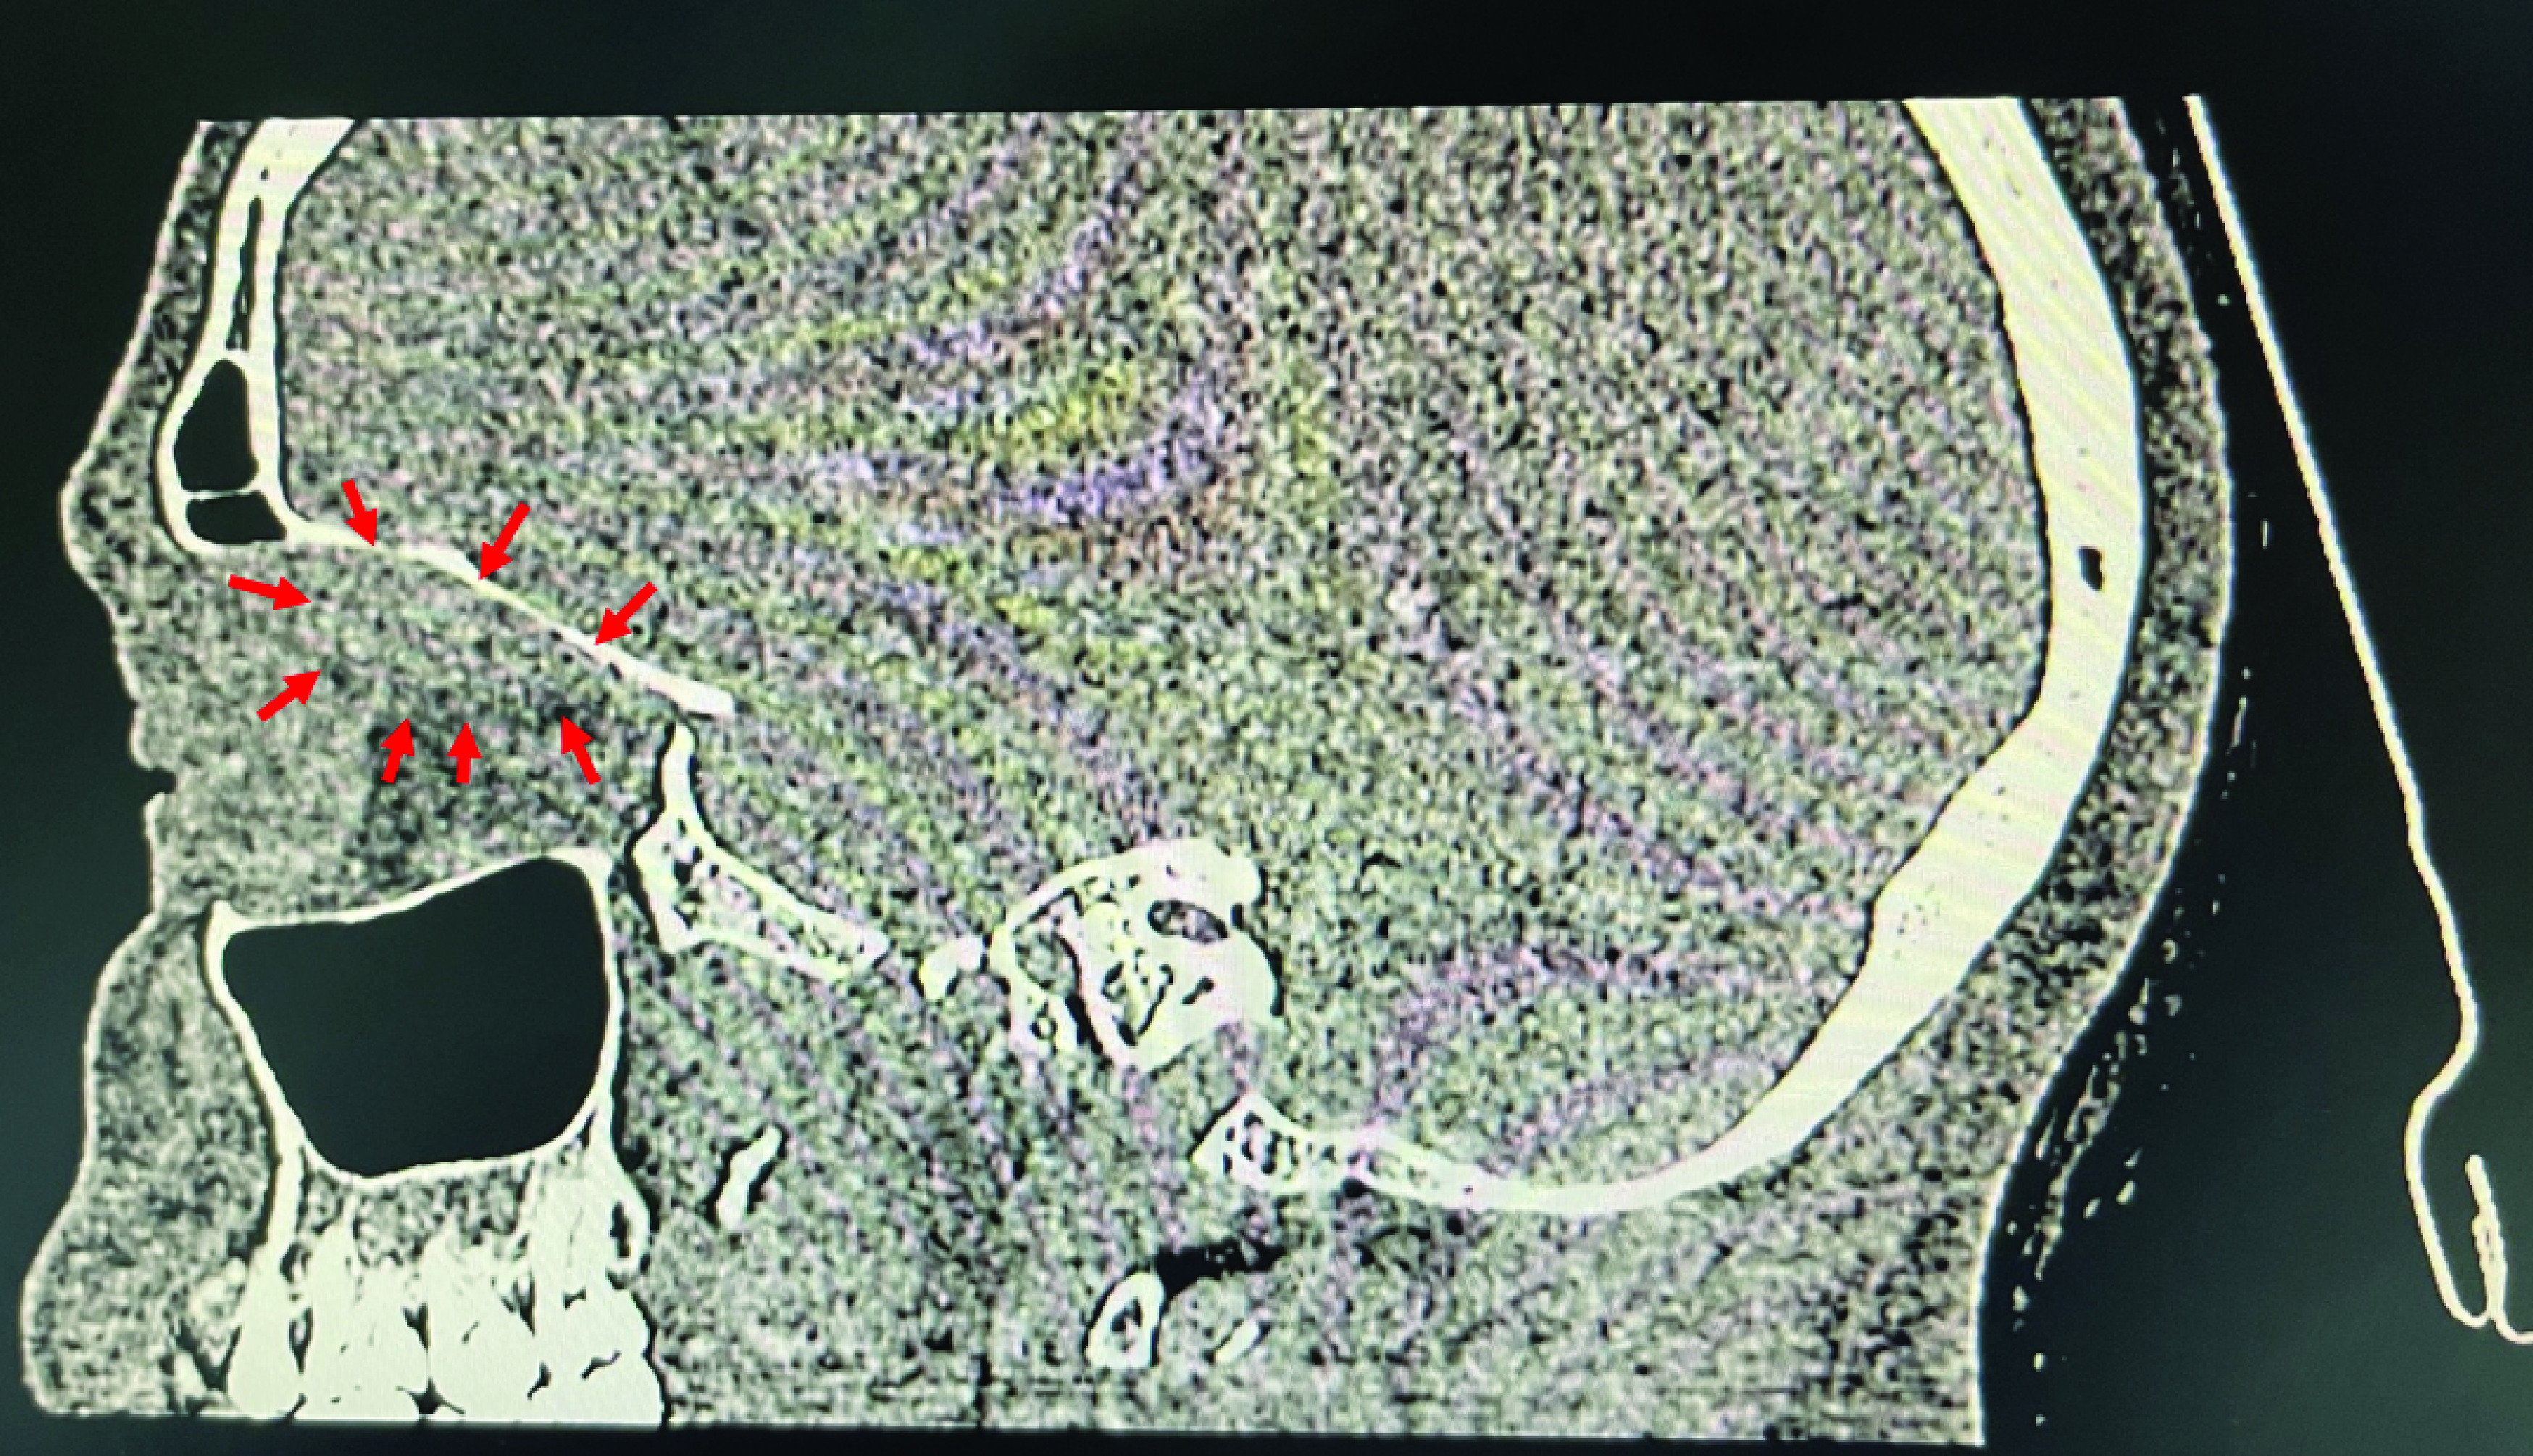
Sagittal computed tomography scan showing a demarcated soft tissue density lesion in the right superior subperiosteal area.
